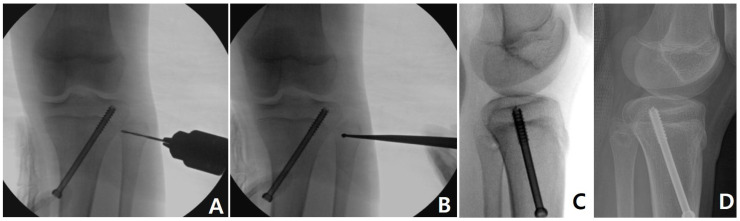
Percutaneous epiphysiodesis of the proximal fibular physis. Through a 0.8 cm skin incision, serial drilling using 2.0~4.4 mm drill bits (A) and additional curettage (B) were performed. Intraoperative (C) and postoperative (D) lateral radiographs showed related defects after the procedures.
